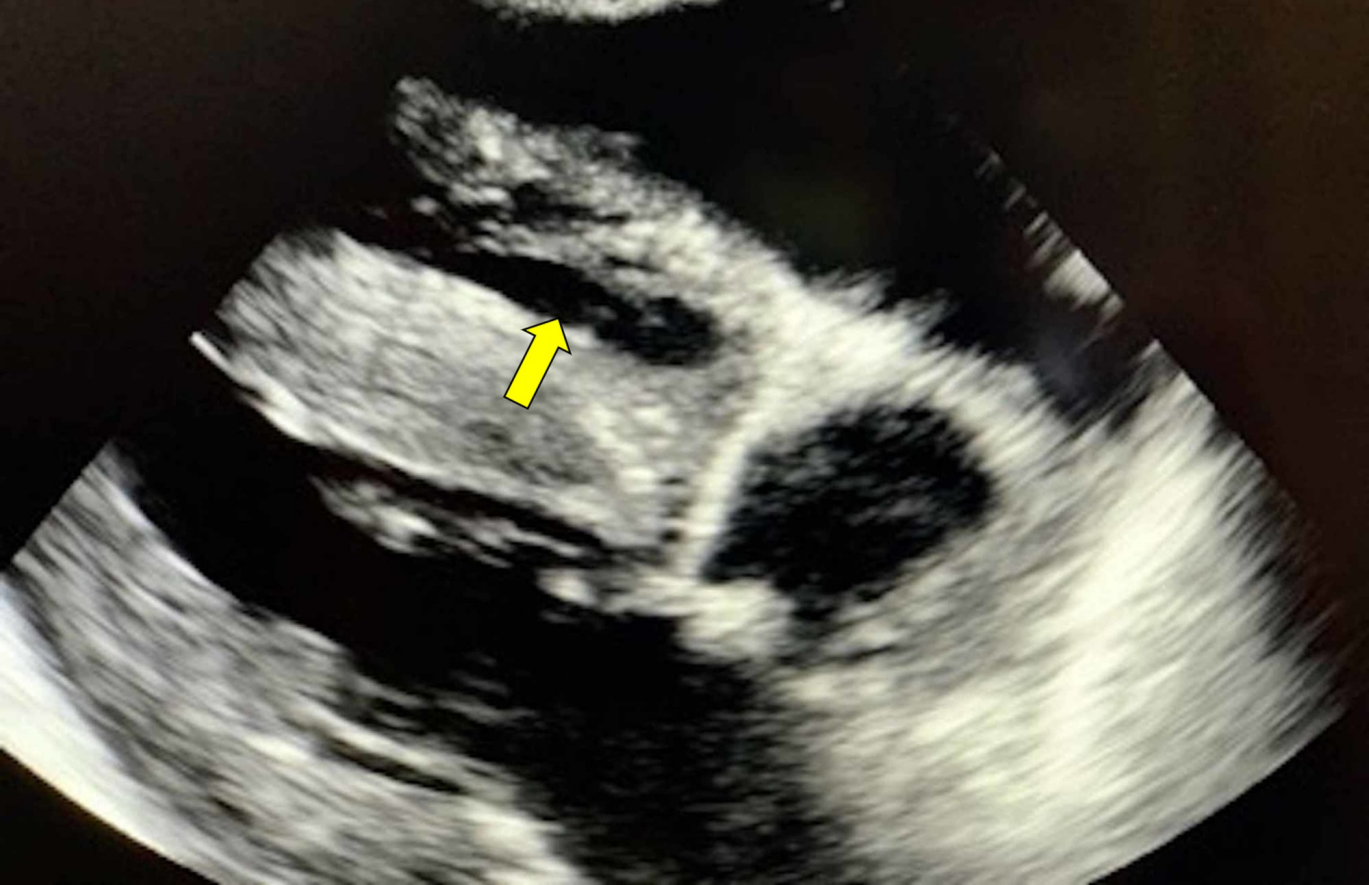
Point of care echocardiogram with parasternal window long-axis (PLAX)-modified view showing severe left ventricular hypertrophy and diastolic right ventricular collapse (arrow indicates collapsing right ventricle in diastole).
